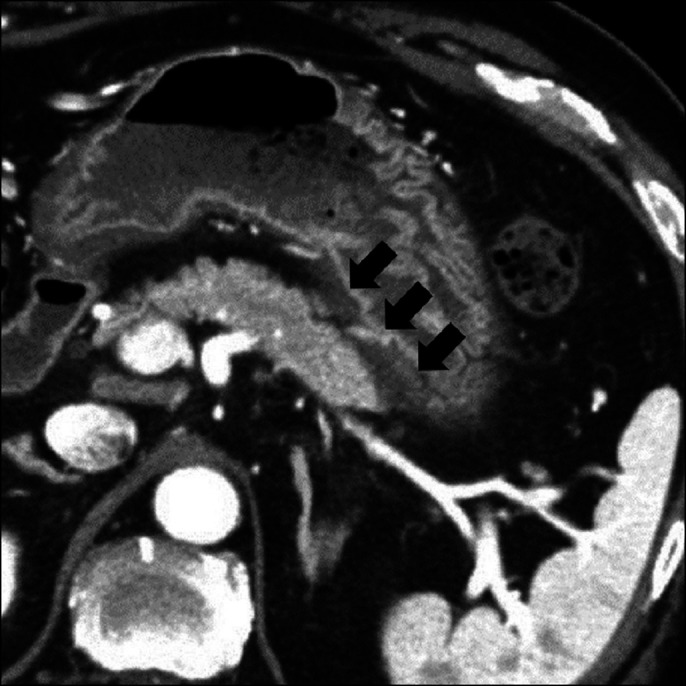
Minimal fluid between the gastric wall and pancreatic body (black arrows) detected around the fine-needle aspiration route on dynamic computed tomography. The black arrows point out minimal fluid correction.
